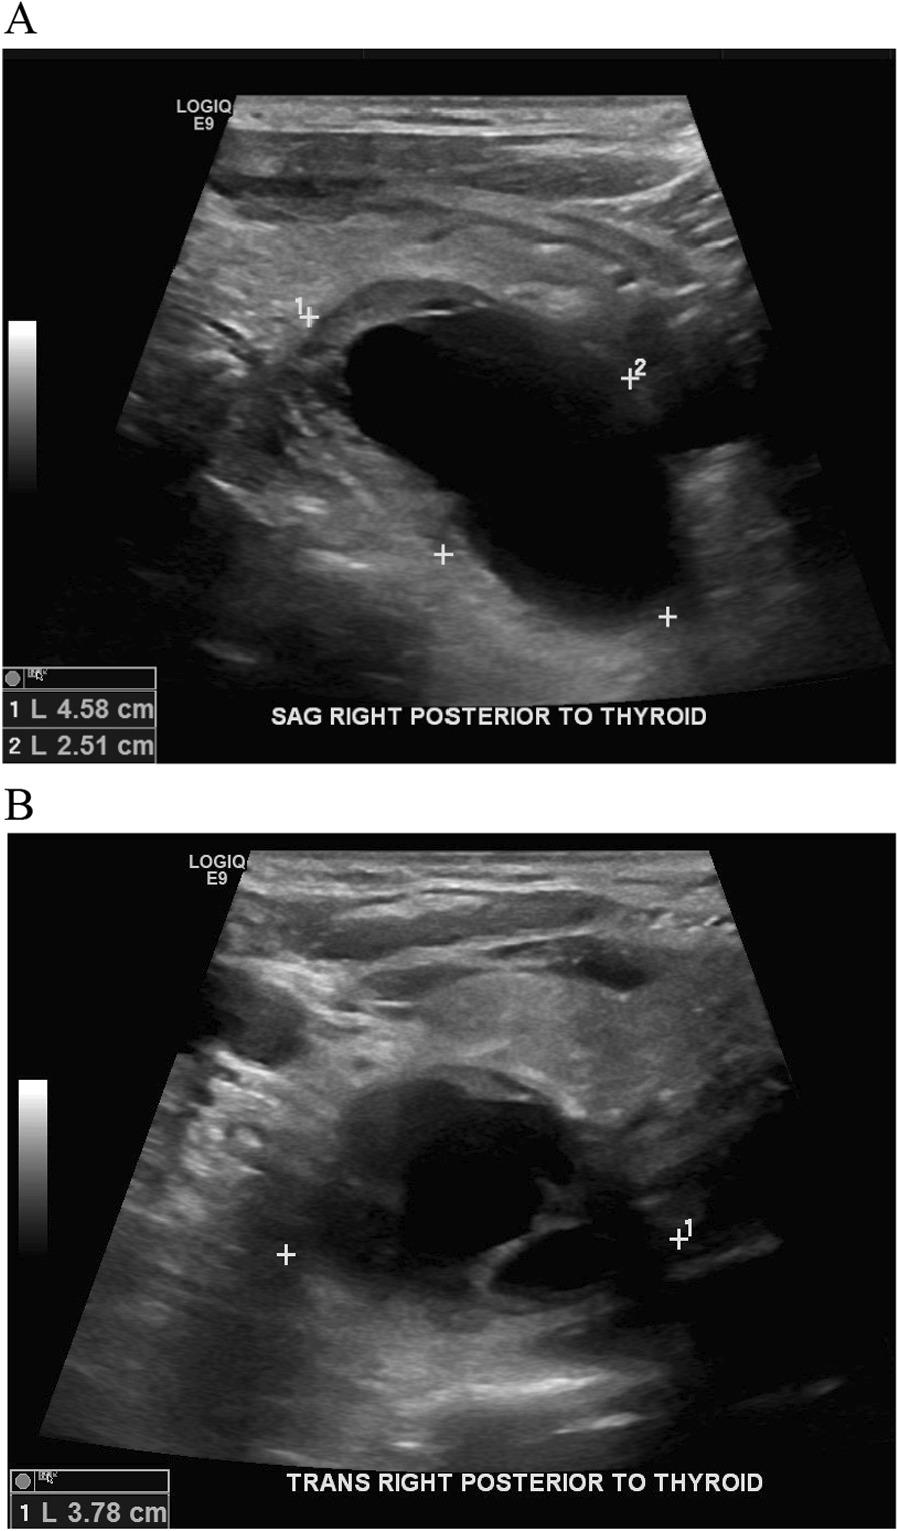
Ultrasound revealing mixed solid/cystic mass posterior to inferior right thyroid measuring 4.6 × 3.8 × 0.5 cm with prominent vascularity in solid rim surrounding cystic portion of mass. A Sagittal view B Transverse view
